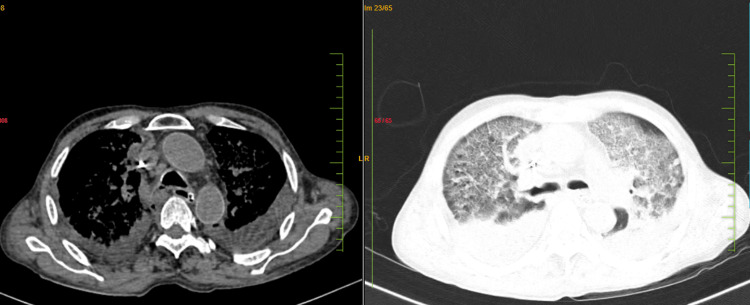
High-resolution computed tomography of the chest shows a non-specific interstitial pattern with patchy areas of consolidation noted in the right upper and left lower lobes with internal cavitations, oblique necrotic areas, and bilateral pleural effusions suggestive of acute respiratory distress syndrome
